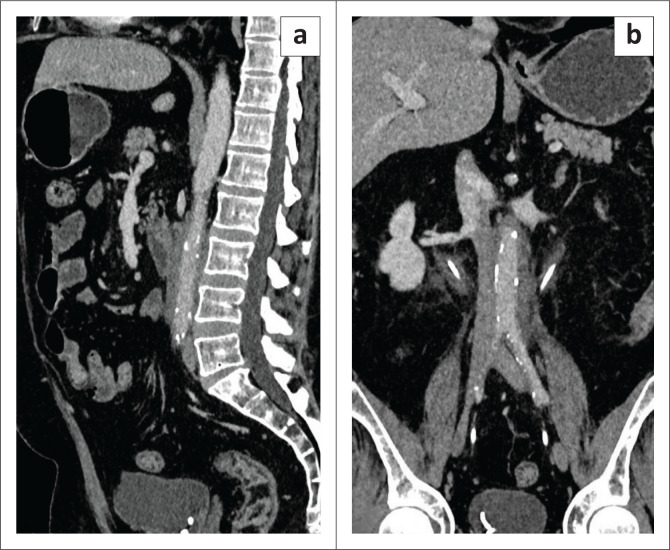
Sagittal (a) and coronal (b) contrast-enhanced CT images show a soft tissue cuff along the abdominal aorta (a–b) and along both upper ureters (b) with ureteric stents in-situ (b).
